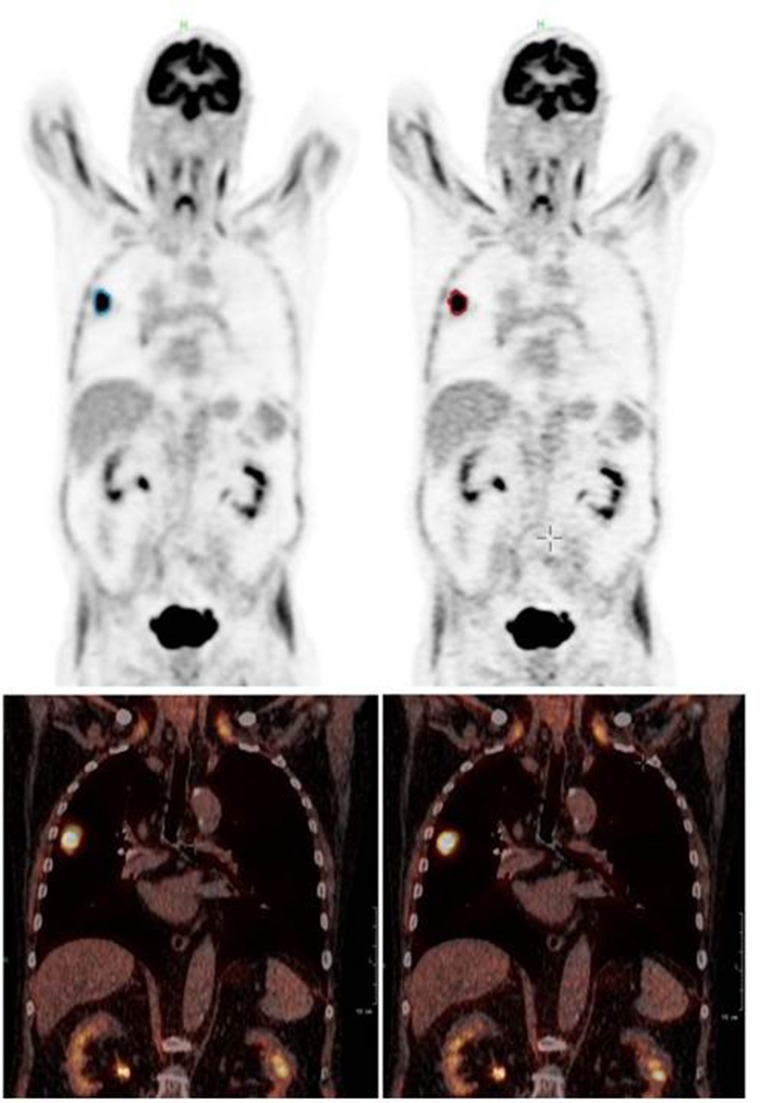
Patient example (Patient 1): coronal PET (top) and fused PET/CT (bottom) of the uncorrected (left) and corrected (right) data set Especially in the fused data set the tumor can be seen less smeared and better fitting to the CT image.
